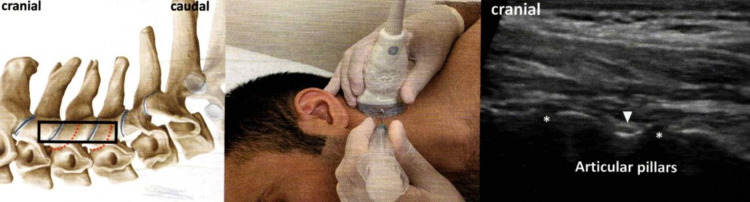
Cervical facet joints injections - lateral approach. The probe (black rectangle) is positioned in a longitudinal plane over the posterolateral neck (left image). In this position, it is possible to target the cervical facet joints using the out-of-plane, anterior to posterior approach, while the patient is in the lateral decubitus position (central image). The corresponding US scan (right image) shows the facet joints (white asterisks) and the medial branches (white arrowhead) as hypoechoic dots located in the grooves of the articular pillars. Originally published in Akkaya N, Constantino J: Upper Cervical Injections. Ultrasound Imaging & Guidance for Musculoskeletal Interventions in Physical and Rehabilitation Medicine. Özçakar L (ed): Edi-ermes, Milan, Italy; 2020. 35-47 [1]. Used with copyright permission from the original publisher.
